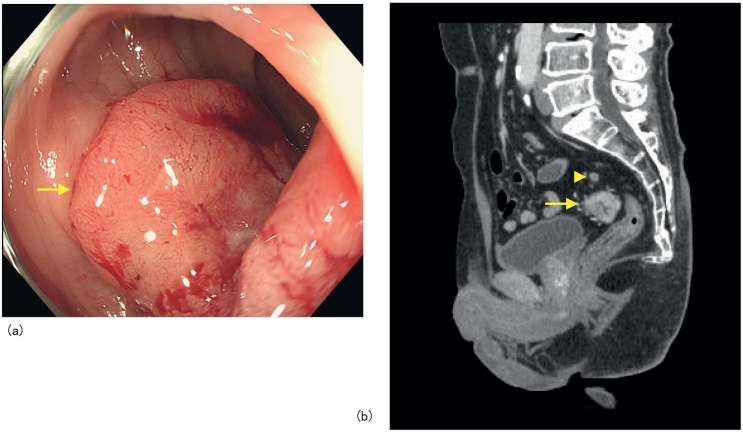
Diagnosis of a primary focus. a) Lower gastrointestinal endoscopy findings: A type 2 rectal tumor was observed (arrow). b) Contrast-enhanced thoracoabdominal CT findings (sagittal section): In the rectum, wall thickening with contrast effects (arrow) and regional lymph node swelling (arrow head) were noted.
